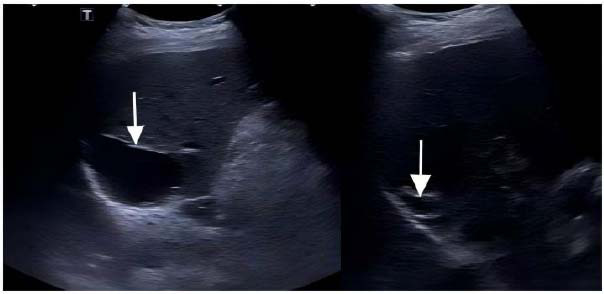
Successful case: Ultrasound image of a 53-year-old man with a hepatic cyst. The initial volume of the liver cyst in the left image was about 330 mL before lauromacrogol hardening treatment. The right image shows that the volume decreased to 9.9 mL (volume reduction rate of 97%) after 12 months of treatment.
